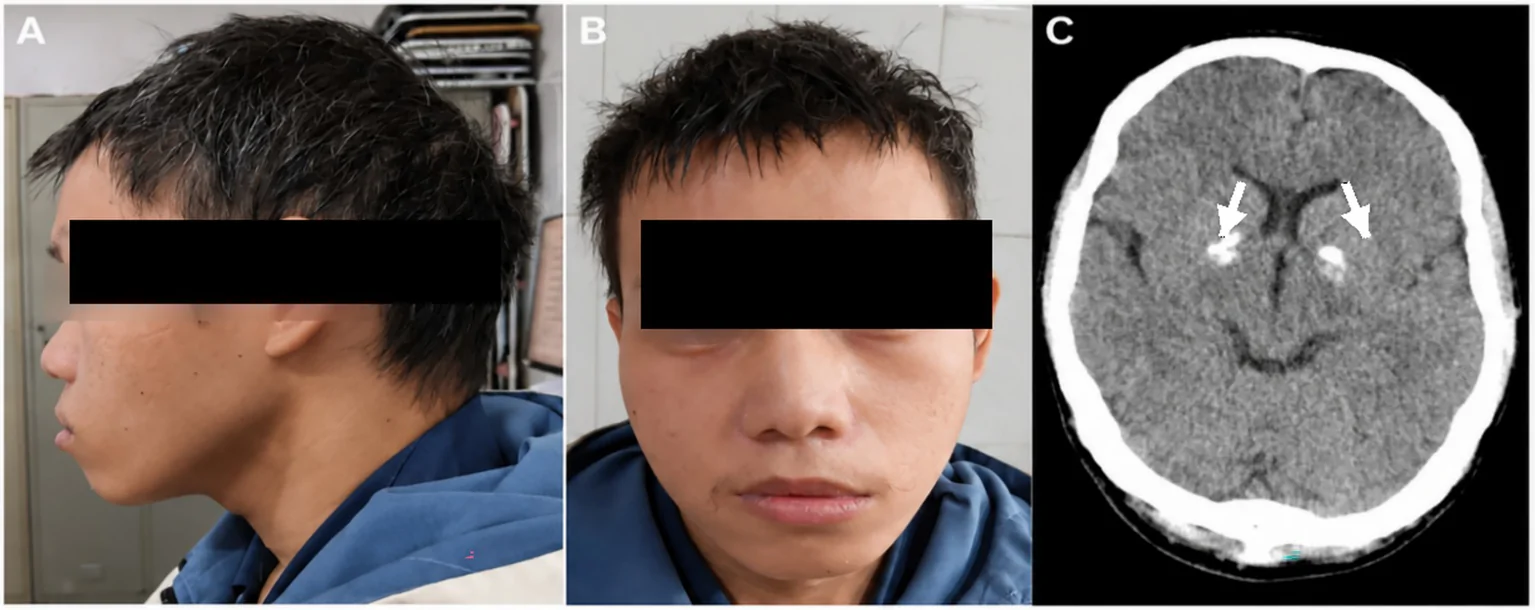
Anonymized facial photographs and basal ganglia calcification in Patient 2. (A) Lateral view showing left facial fullness. (B) Frontal view demonstrating facial asymmetry. The eye region has been masked to reduce identifiability. (C) Axial non-contrast head CT showing bilateral symmetric basal ganglia calcification (arrows). CT, computed tomography.
